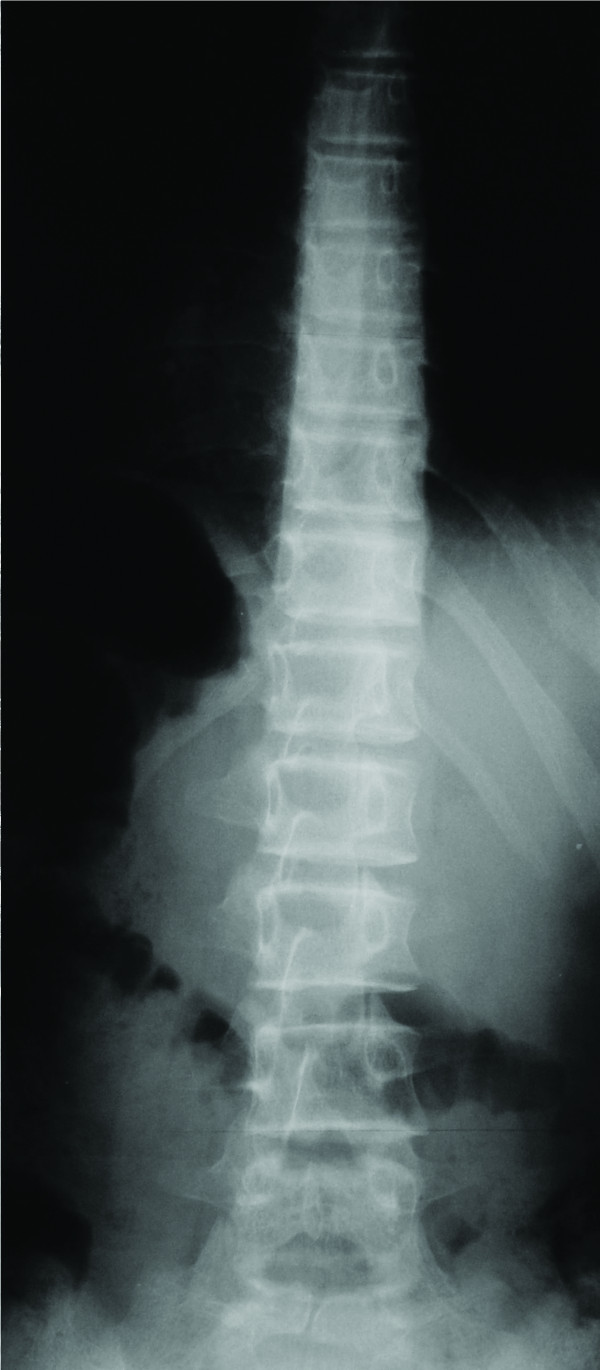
Standing posterior-anterior radiograph of the patient's spine 12 months after her initial examination (Visit 2). The vertebral rotation has significantly deteriorated leading to the decision to fit the patient with a modified thoracolumbar Boston Brace.
